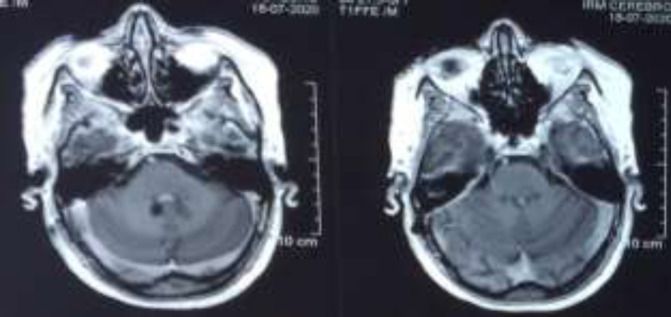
MRI findings after glucocorticoids-based treatment
